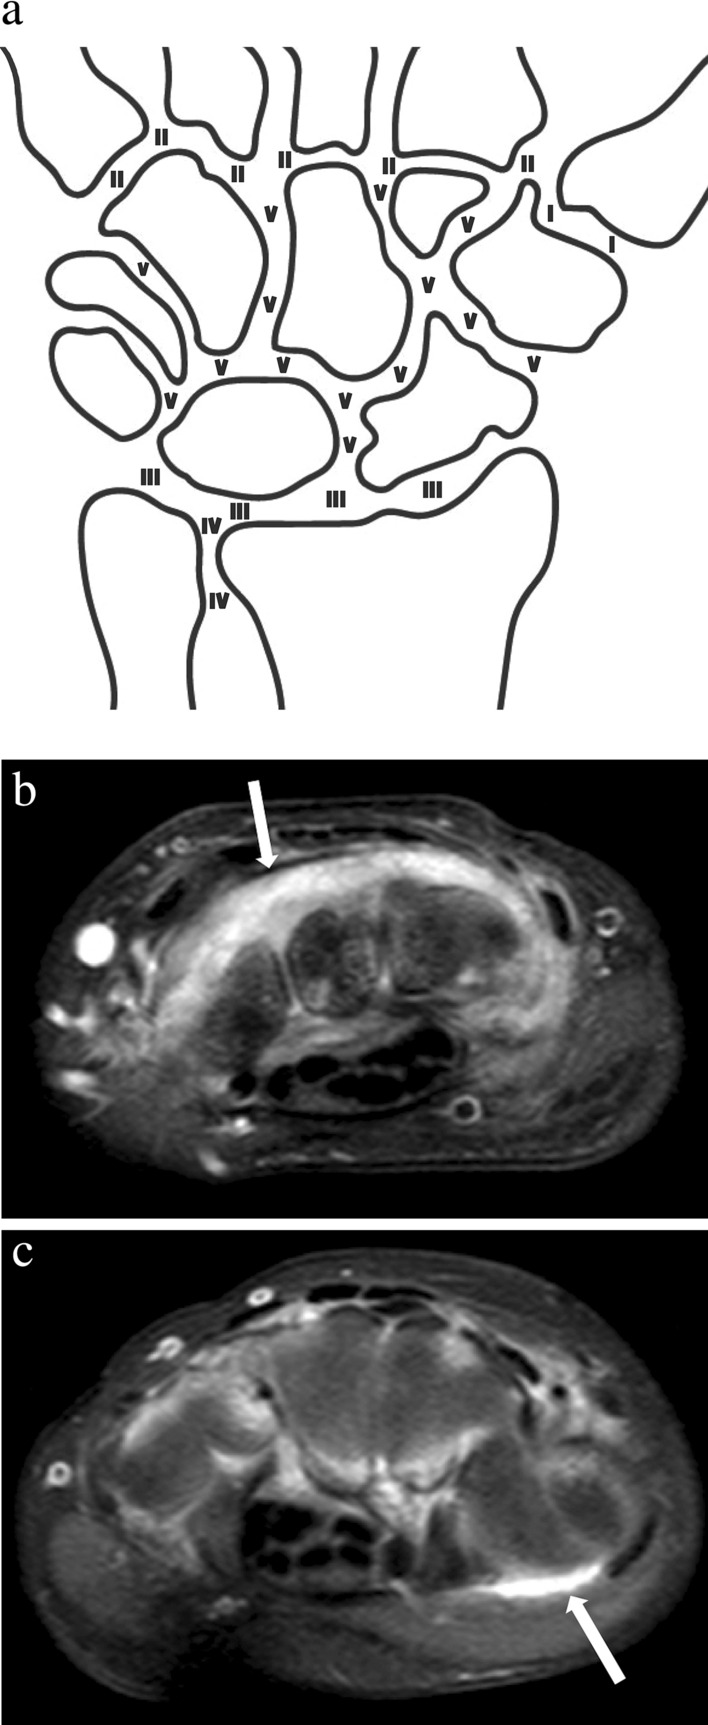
a Anatomical scoring sites of the synovium. (I) First carpometacarpal recess, (II) 2nd–5th carpometacarpal joints, (III) Radiocarpal joint, (IV) Distal radioulnar joint, (V) Mid-/inter-carpal joints. Example of a contrast-enhanced MRI image of b severe synovial thickening in the wrist of a 17-year-old female JIA patient, and (c) severe synovial enhancement in the wrist of a 9-year-old female JIA patient
